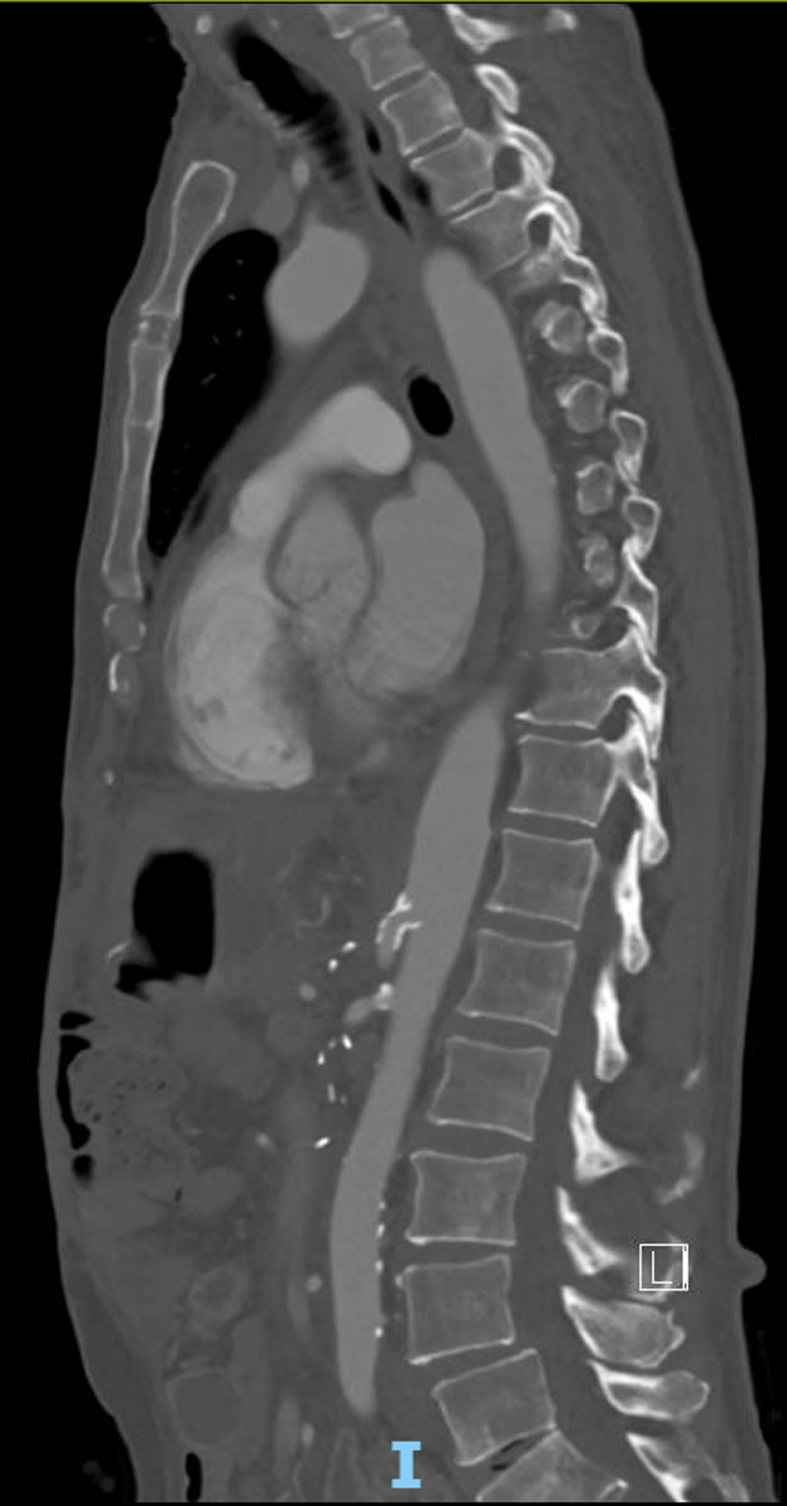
Computed tomography angiography (CTA) at 6 months postoperatively of the abdomen and pelvis showing a patent celiac artery stent extending proximally 1 to 2 mm into the abdominal aorta.
